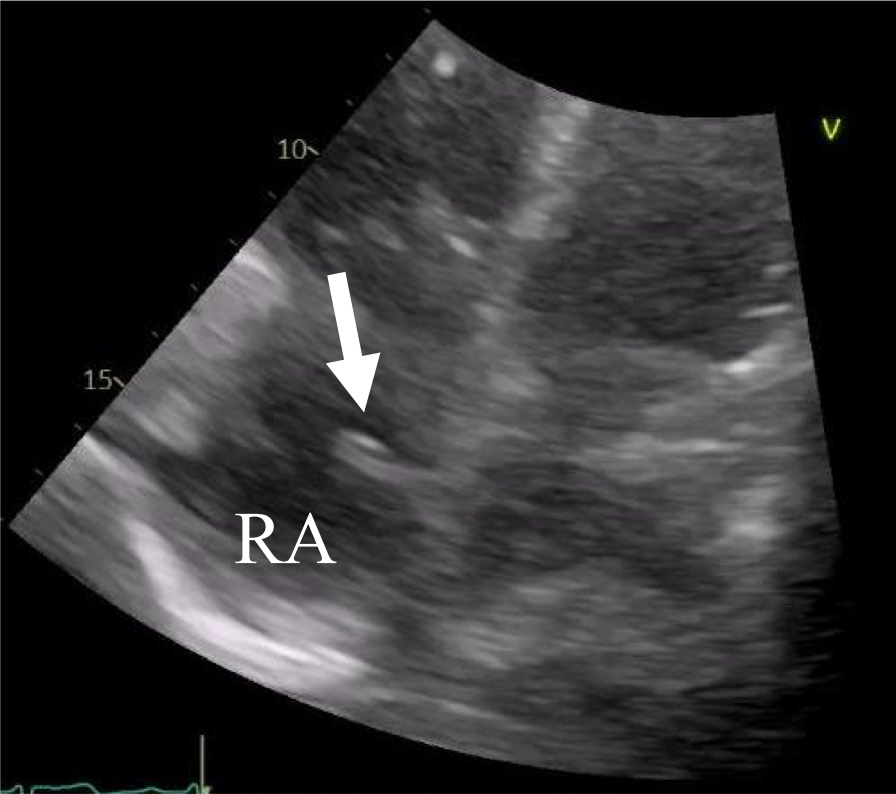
Transthoracic echocardiography showing a thrombus lodged in the foramen ovale (arrow: thrombus, RA: right atrium)
